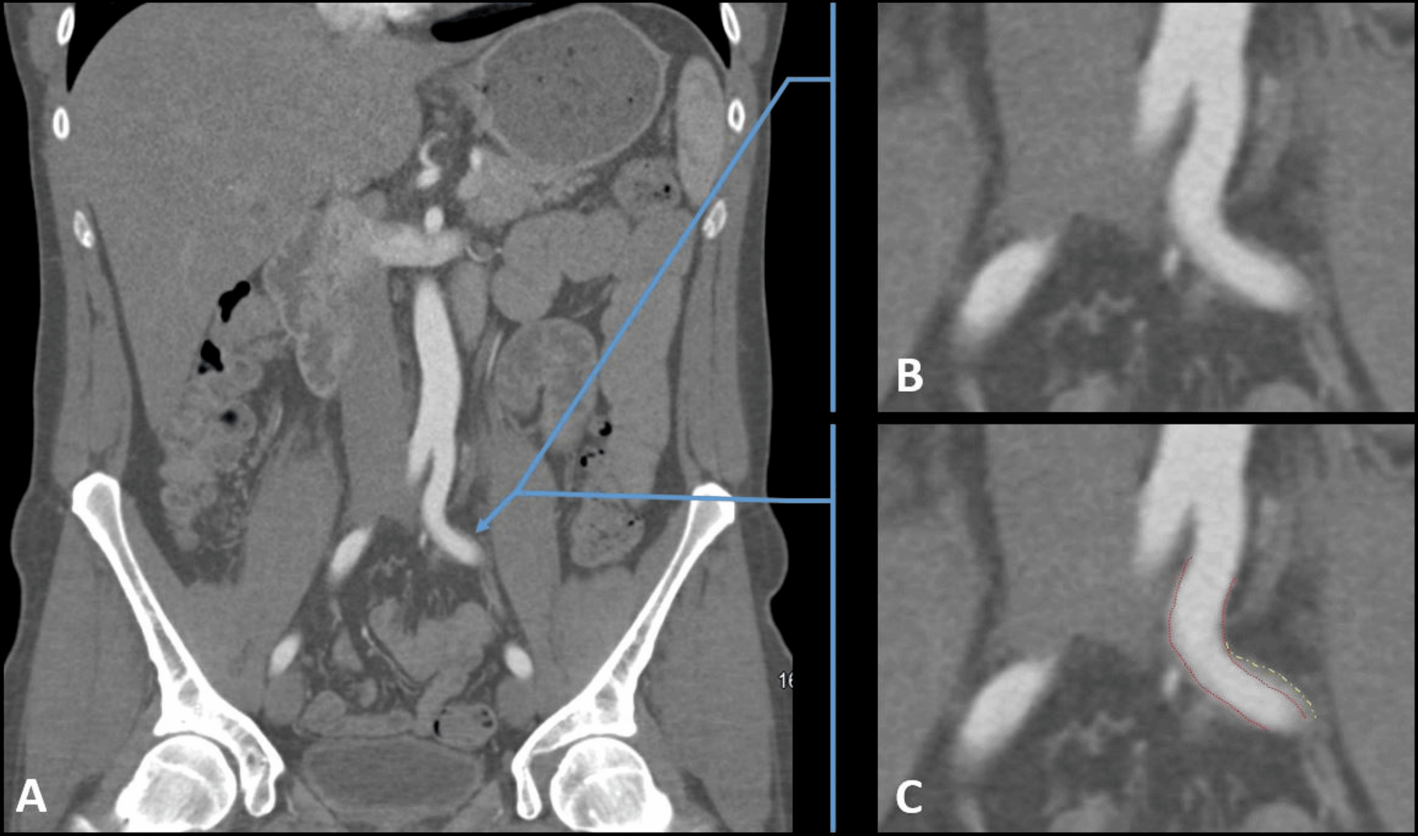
CT angiography of the pelvis in the coronal plane Contrast-enhanced CT imaging of the pelvis in the early arterial phase provides evaluation of the arteries. Specifically, in this cross section, the common iliac artery (CIA) is shown bilaterally relative to surrounding pelvic viscera in a non-magnified view (A). (B and C) Cropped magnified coronal images created from (A). (C) Annotations of the normal vessel (red perforated oval) versus the abnormal fibrotic change surrounding the vessel (yellow perforated oval).  The CIA on the left is pathologic, whereas the CIA on the right is normal (A-C).
